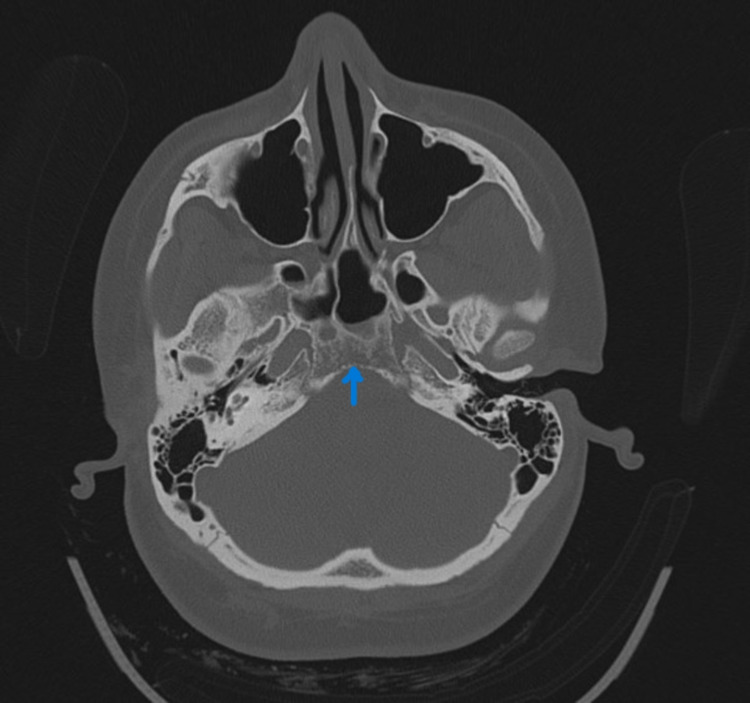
Axial CT at the skull base reveals erosion of basisphenoid bone.
